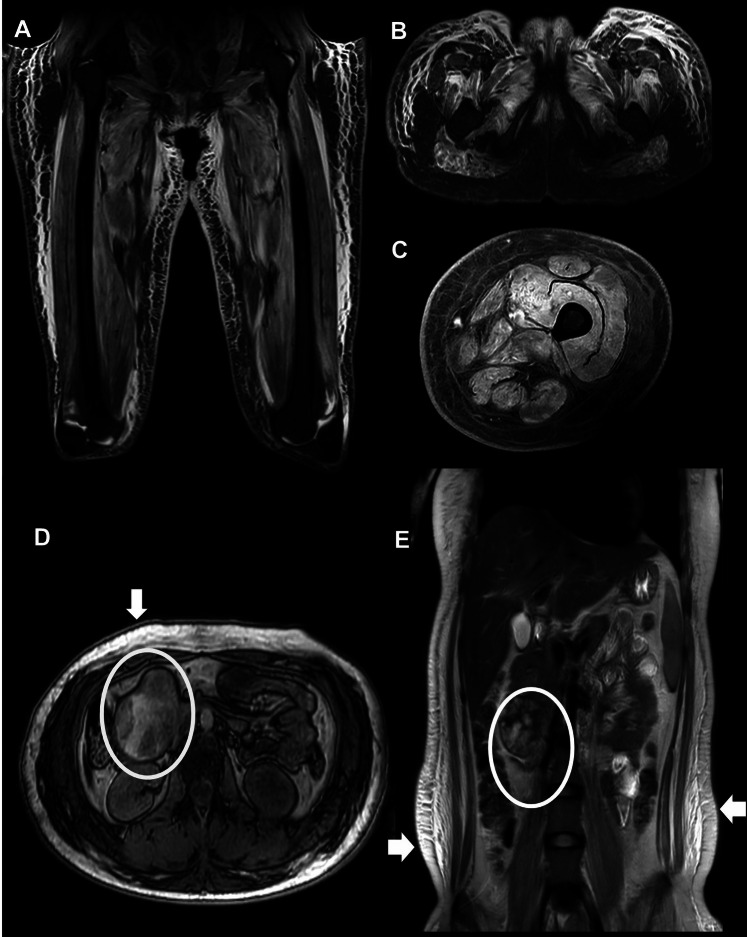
Non–contrast-enhanced MRI of the thighs. A. Coronal view. B-C. Axial view. Asymmetric and diffuse muscle edema involving the anterior, medial, and posterior compartments. Contrast-enhanced abdominal MRI. D. Axial view. E. Coronal view. Solid–cystic mass in the pancreatic head and uncinate process (white circles) and generalized subcutaneous edema (white arrows)
